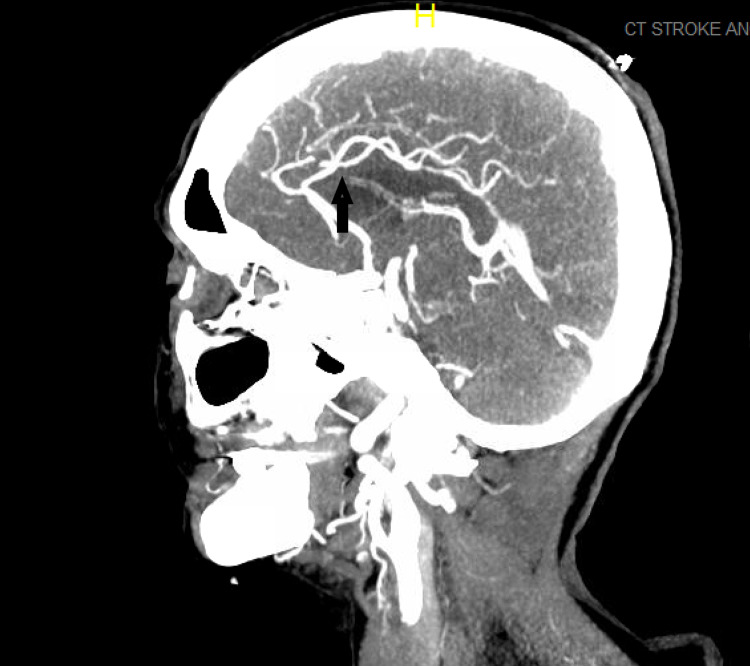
CT angiogram of head and neck showing the sagittal view of the middle cerebral artery occlusion.
